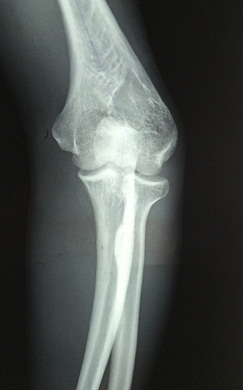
Radiographie de face montrant la déformation en varus du deuxième patient
